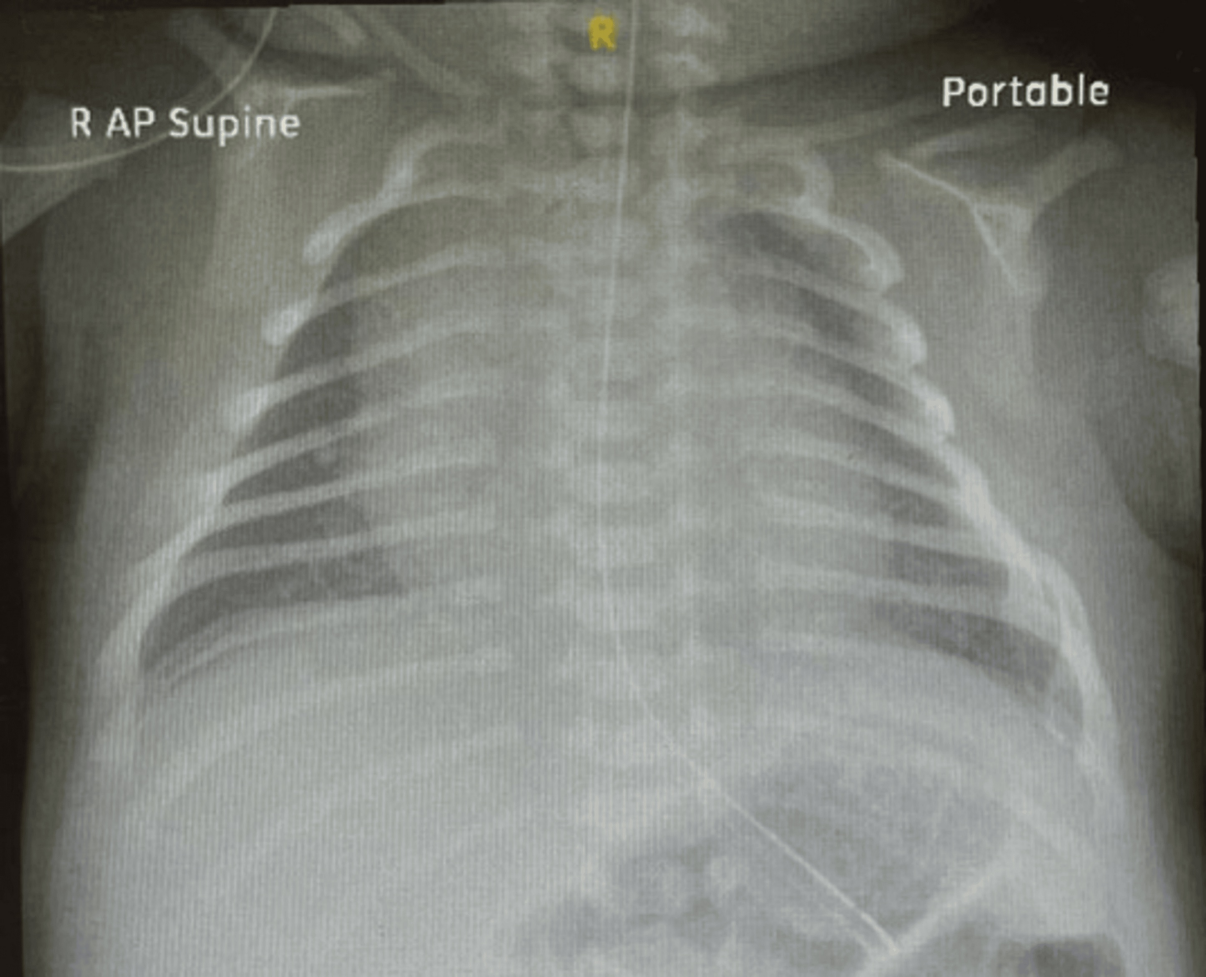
Initial Bilateral Clavicle X-ray.
